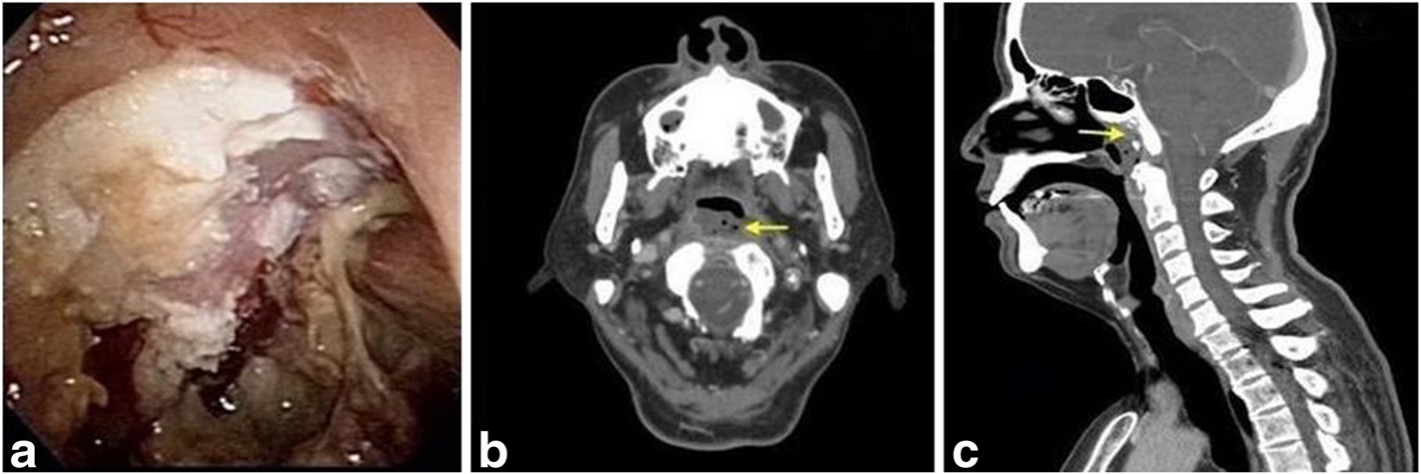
a Nasopharyngoscope shows crusts and pus accumulated on the nasopharynx, and sequestration of the skull base as a result of osteoradionecrosis. b & c CT of head and neck, axial and sagittal cuts, arrows show evidence of osteoradionecrosis of the skull base
